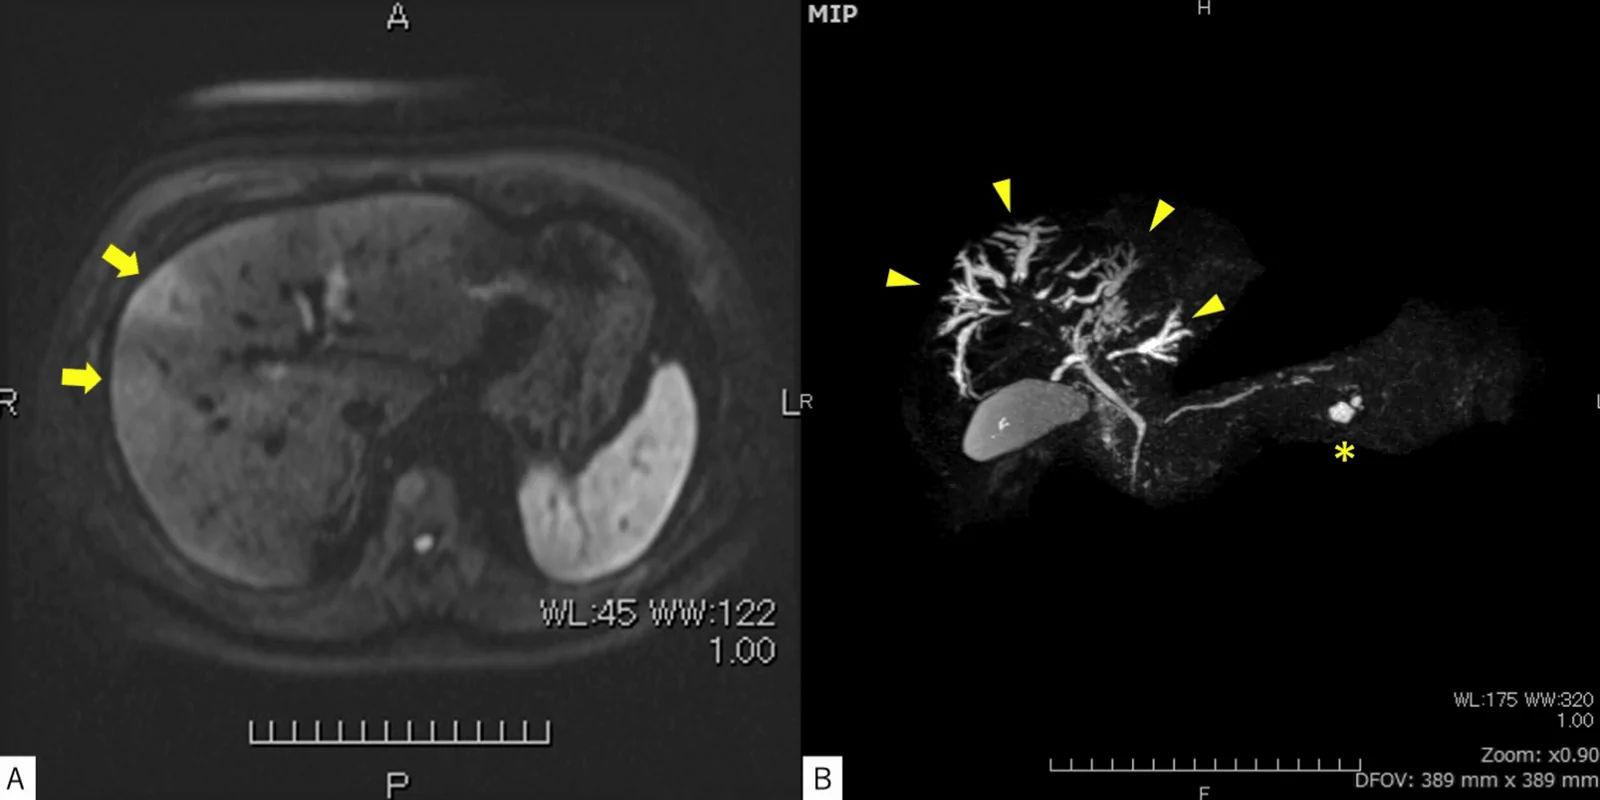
Magnetic resonance imaging and MRCP findings at the time of relapse (Month 32) (A) Axial diffusion-weighted imaging revealing high signal intensity along the peripheral intrahepatic bile ducts in the right hepatic lobe (arrows), indicating active inflammation. (B) Maximum intensity projection MRCP image demonstrating multiple smooth strictures in the intrahepatic and extrahepatic bile ducts, accompanied by upstream dilation (arrowheads). A cystic lesion is also clearly noted in the pancreatic tail (asterisk) MRCP: magnetic resonance cholangiopancreatography
